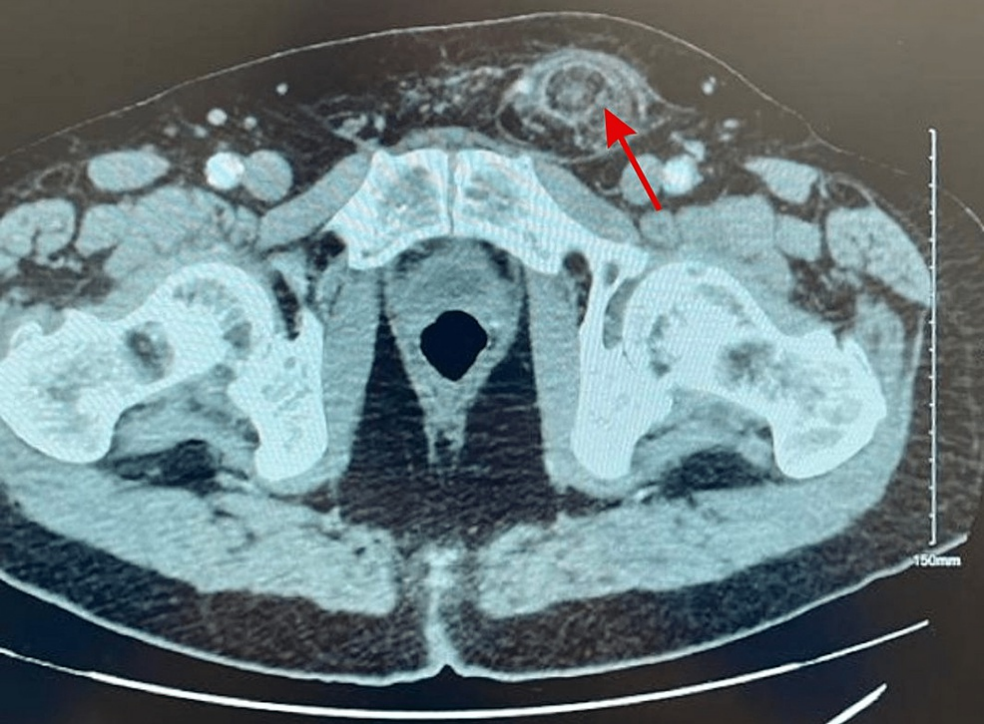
CT scan of the pelvis and lower abdomen. Axial plane of the CT scan. The red arrow indicates the left inguinal hernia.
